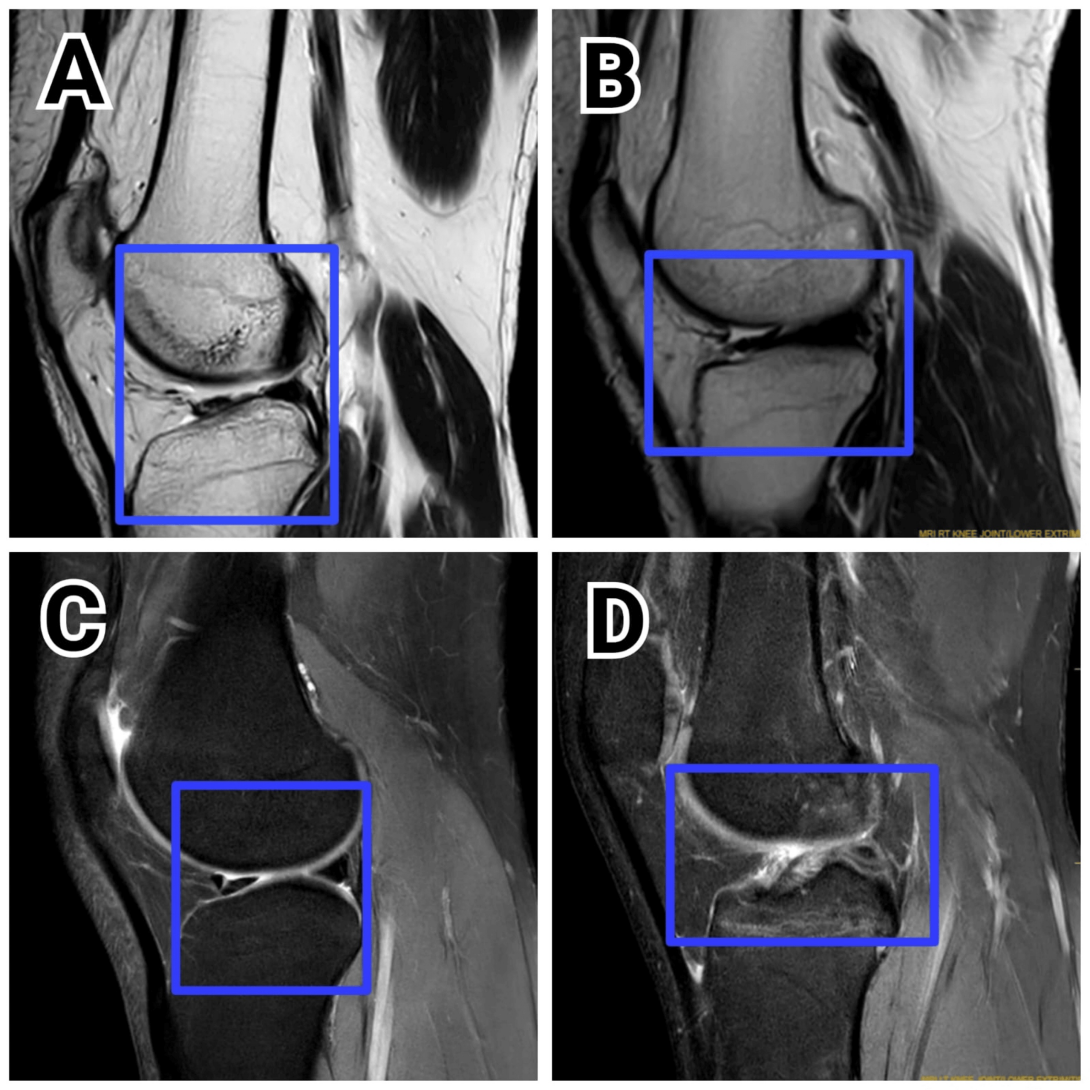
Preoperative MRI of all the four patients A: Patient 1; B: Patient 2; C: Patient 3; D: Patient 4 The highlighted region shows a tear in the anterior cruciate ligament.
